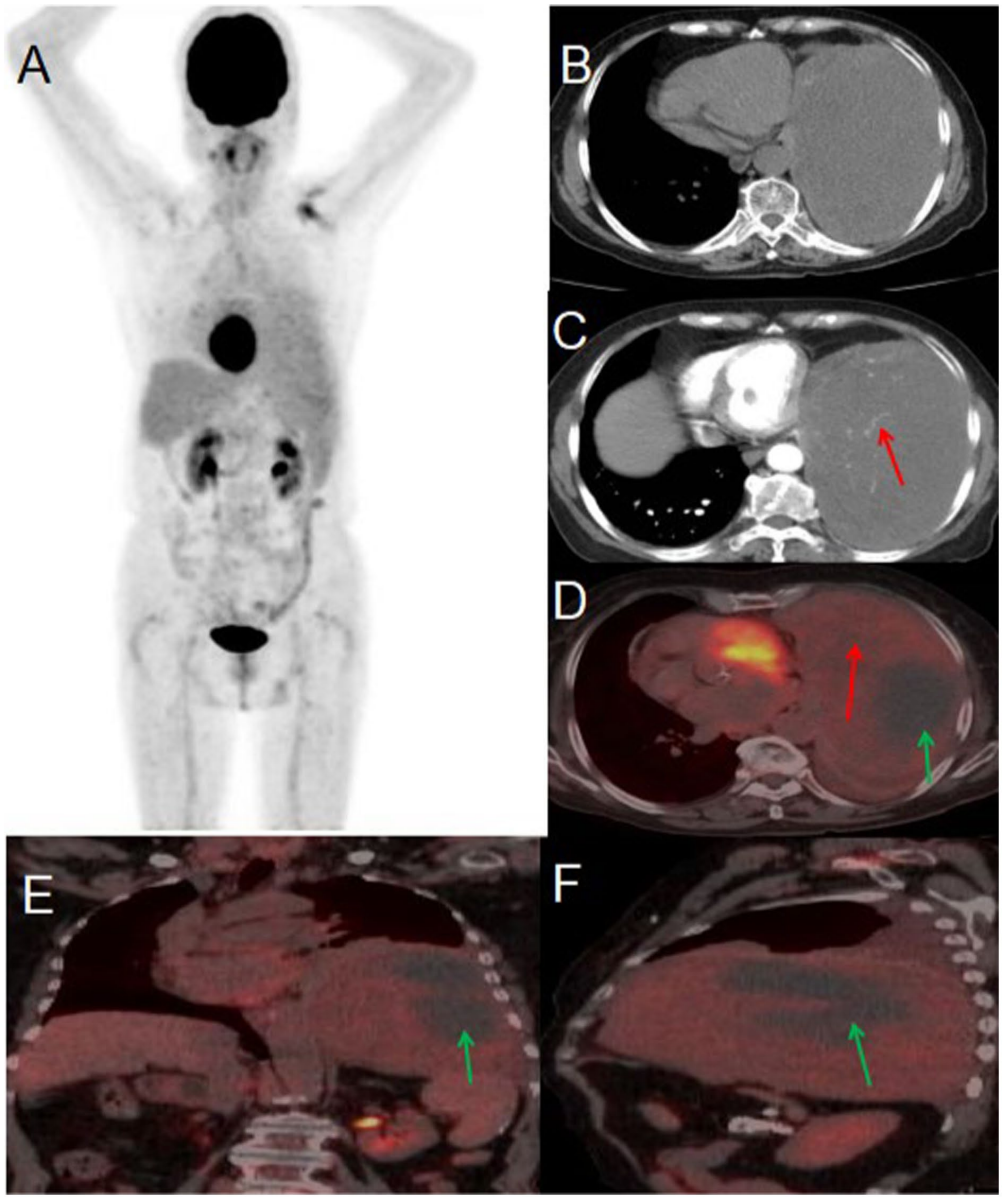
Male, 80 years old, benign primary pulmonary solitary fibrous tumors. (A) Whole body MIP. (B) Axial, non-contrast-enhanced CT. (C) Axial, enhanced CT-arterial phase. (D) Axial fusion. (E) Coronal fusion. (F) Sagittal fusion. PET/CT imaging revealed a large, mixed-density soft tissue mass in the left lung with necrosis and indistinct boundaries, measuring approximately 97 × 157 mm at its largest dimension. The mass exhibited slightly increased heterogeneous FDG uptake, with an SUV_max_ of 2.3 (red arrow, D), while no FDG uptake was observed in the low-density necrotic area (green arrow, D–F). The contrast-enhanced CT scan showed an irregular, large soft tissue mass in the left lung with heterogeneous density and visible necrosis. The enhanced scan demonstrated heterogeneous enhancement with prominent vascular shadows (red arrow, C) and local encapsulation.
